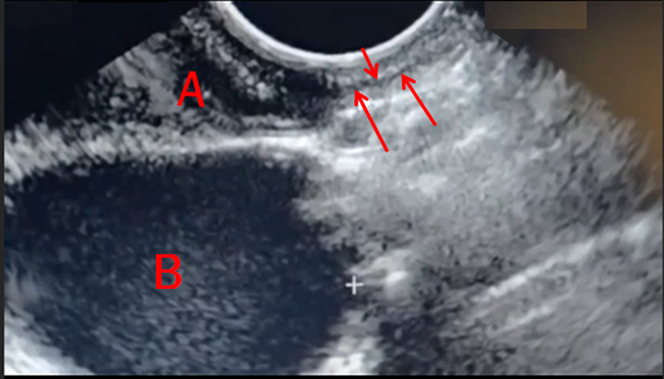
Endoscopic ultrasonography image showing a subepithelial lesion (A) originating from the fourth layer, specifically the circular muscle layer of the muscularis propria (indicated by *arrows*). The lesion is in close proximity (only 1 mm) to the aorta (B), posing a significant challenge because of the patient's comorbidities and the lesion's size.
